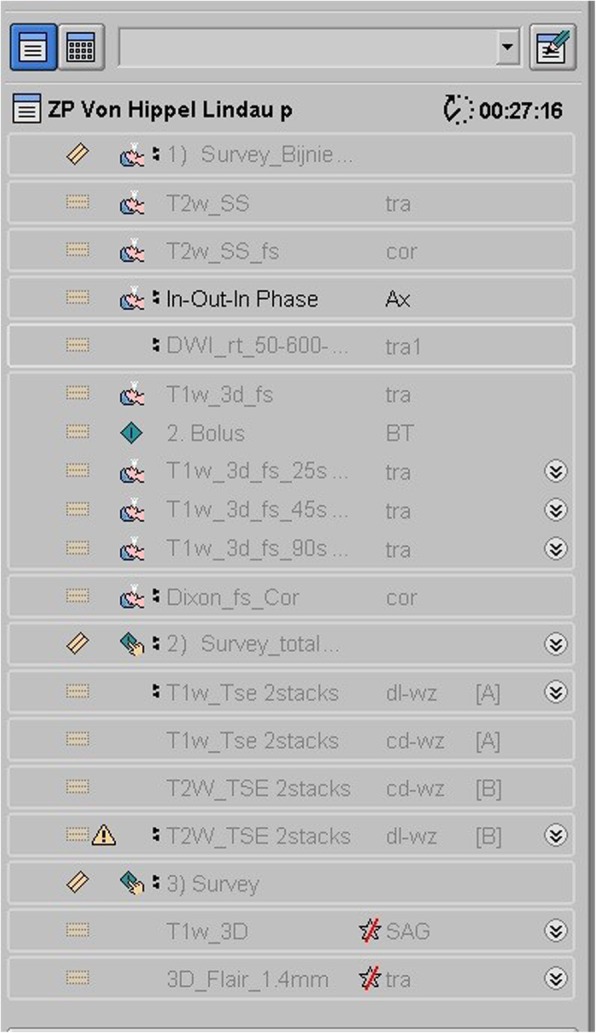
MRI protocol. Screenshot of the examination card we use for our screening protocol, from the MR examination console. Notice the examination time of 27 min and 16 s mentioned on top of the list (time calculated without reconstruction time needed in between sequences)
